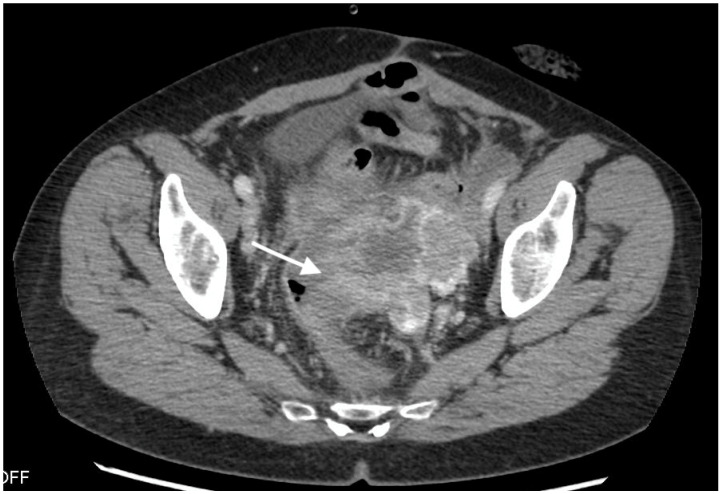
Images from 58-year-old patient with carcinosarcoma of the ovary and recurrence in the rare form of giant cell carcinoma. The fistula between tumor and rectum (arrow), CT post-contrast image in venous phase obtained in the axial plane, shows pelvic tumor and adjacent intestine, fatty tissue between the tumor and intestine vanished as indirect sign of the fistula.
